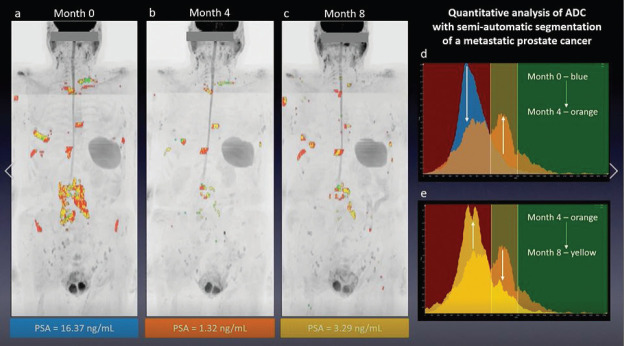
Metastatic PC staging with WB-MRI. A 70-year-old male with high-risk PC (PSA 28 ng/mL, Gleason score (GS) 4+4) seen in (a): coronal inverted grey-scale MIP of b-900 diffusion-weighted images and (b–d): axial b-900 diffusion-weighted images b-900 images from WB-MRI performed for staging. In the ‘at-a-glance’ disease assessment facilitated by the MIP, WB-MRI shows a clinical pattern of type N1 M1b, involving (b): regional lymph node metastasis (c): a non-regional lymph node metastasis and (d): bone marrow disease.
